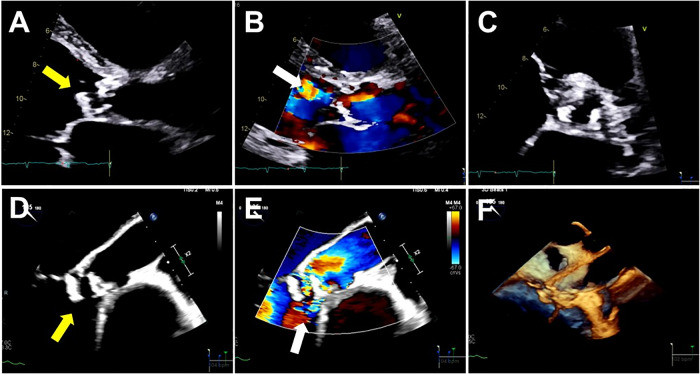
Transthoracic and transesophageal echocardiography of aortic-valve infective endocarditis. (A) Transthoracic parasternal long-axis view showing a large mobile aortic-valve vegetation (yellow arrow). (B) Color Doppler image showing severe eccentric aortic regurgitation (white arrow). (C) Additional transthoracic view demonstrating bicuspid aortic-valve morphology. (D) Transesophageal view showing a flail aortic leaflet with attached vegetation (yellow arrow). (E) Color Doppler transesophageal view confirming severe eccentric aortic regurgitation (white arrow). (F) Three-dimensional transesophageal echocardiography showing the abnormal bicuspid aortic valve.
